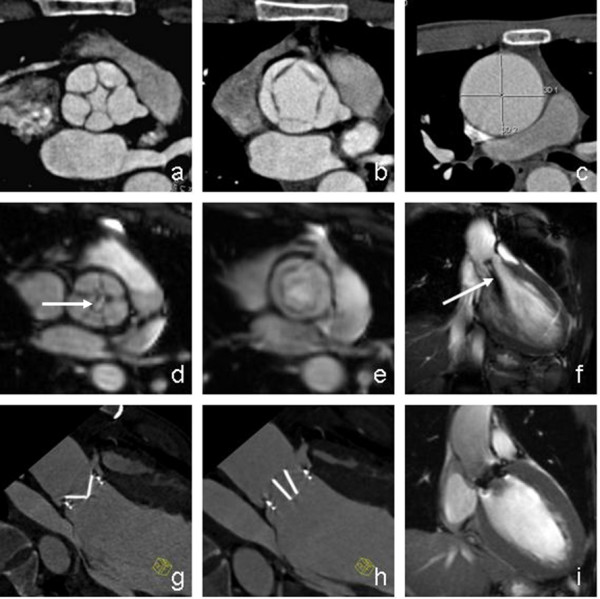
CT and CMR of quinticuspid aortic valve pre- and post-operative examination. (a) Dual source CT revealed malformed aortic valve in diastolic phase. (b) Dual-source CT showed malformed aortic valve in systolic phase. (c) Dilated ascending aorta. (d) CMR revealed malformed aortic valve in diastolic phase. (e) CMR showed malformed aortic valve in systolic phase. (f) Regurgitation in diastolic phase revealed as signal void (arrow). (g) Dual source CT showed mechanical valve in systolic phase. (h) Dual source CT showed mechanical valve in diastolic phase. (i) There is no regurgitation in diastolic phase.
